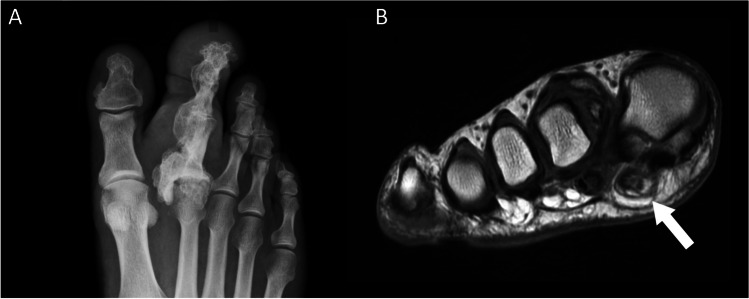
Radiographs of the right foot in the patients with (A) NF1 and (B) macrodystrophia lipomatosa with focal gigantism of the (A) third and (B) second toes. The patient with NF1 (A) has a long third toe with little soft tissue hypertrophy but they do also have a dysplasia of the 4th metacarpal. The patient with macrodystrophia lipomatosa has hypertrophy of the bone and overlying soft tissues in a sclerotomal distribution along the medial digital nerve
